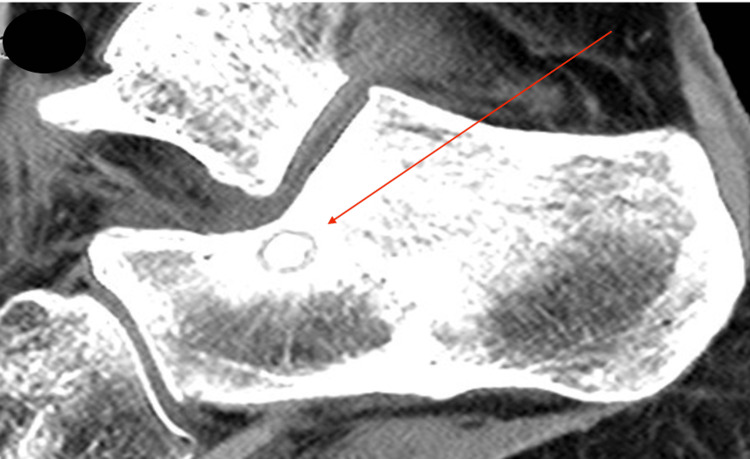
Sagittal view of computed tomography of calcaneus The red arrow depicts a circular area (nidus) surrounded by dense bone in the anterior calcaneal region.
